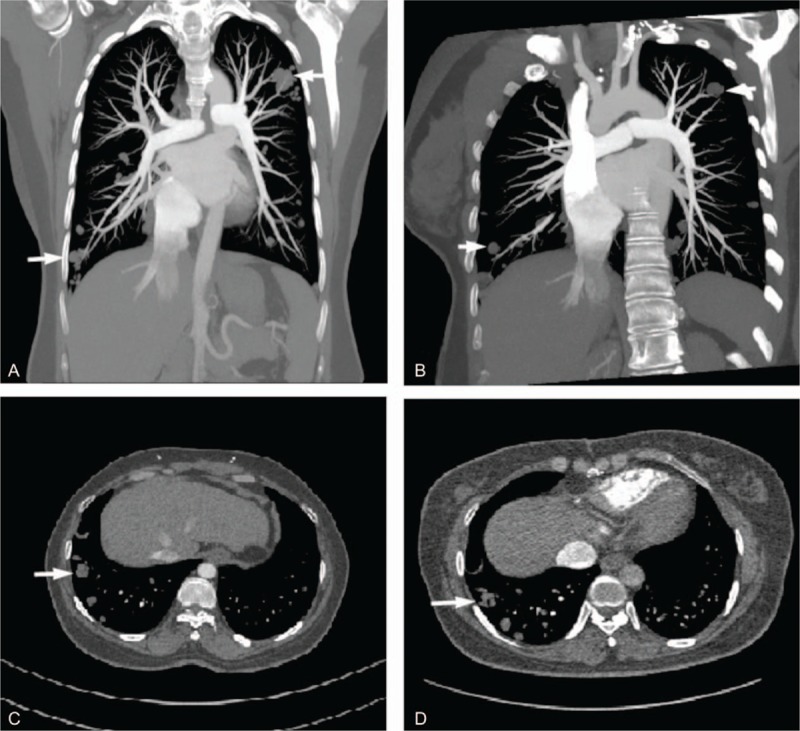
Computed tomography images of case 1 were shown. Multiple round-shaped nodules (arrows) with different sizes were randomly distributed in bilateral lung fields in coronary plane (A), sagittal plane (B), and axial plane (C) in April 2014. Multiple nodules in bilateral lung fields changed little (D) in September 2014.
